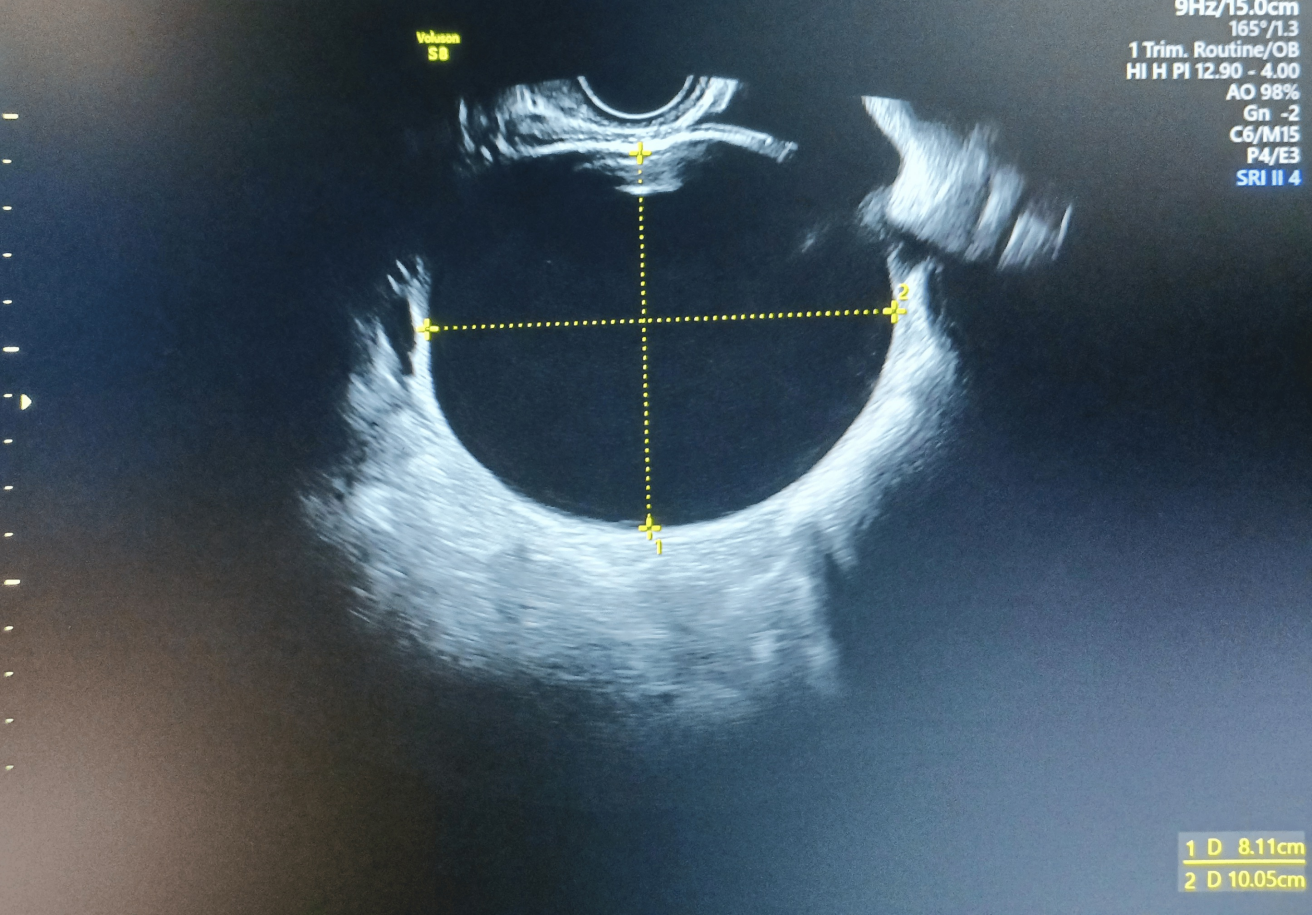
Transvaginal ultrasound exam in the first trimester of pregnancy reveals an ovarian mass.
